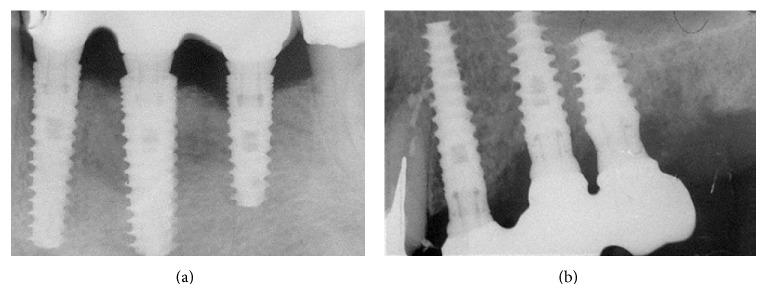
The X-ray control after final prosthesis.
